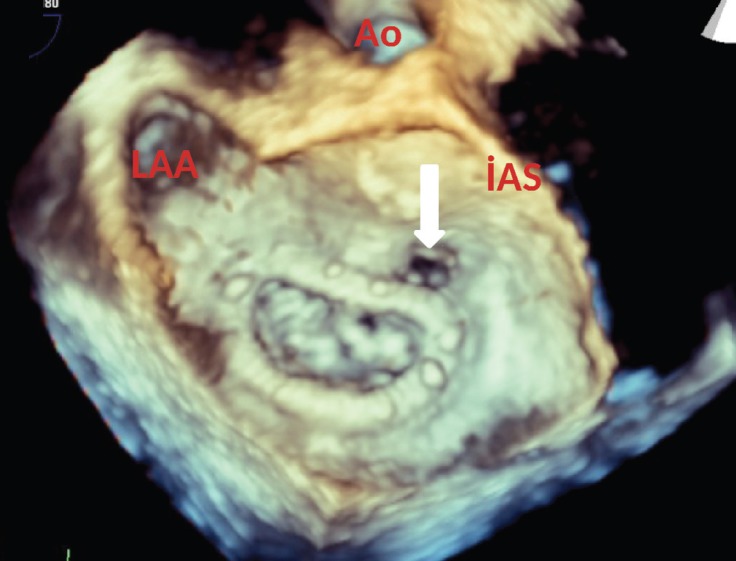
Three-dimensional transesophageal echocardiography, showing the anterior mitral annulus para-ring hole in the anteromedial segment (arrow).
